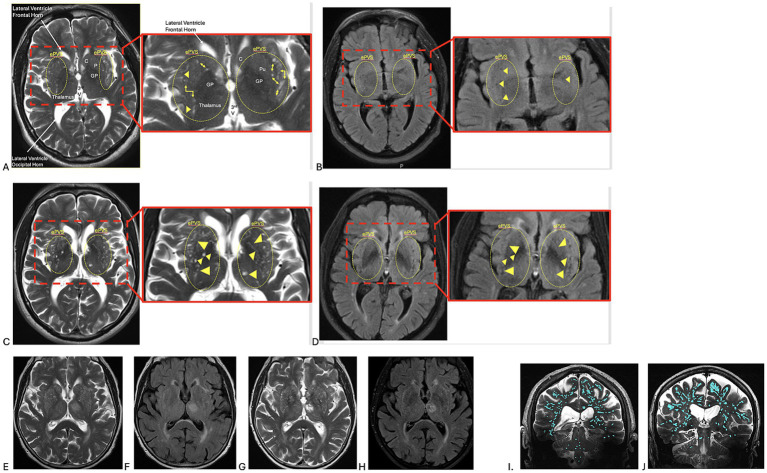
(A,B) Longitudinal MRI of a 65-year-old male individual with Alzheimer’s dementia on 1.5 T MRI. (A) Axial T2 and (B) axial FLAIR images at baseline. A total of 10 years later, the same patient is shown on (C) axial T2 and (D) axial FLAIR images, now demonstrating enlarged perivascular spaces (ePVSs) (highlighted within yellow dotted lines and indicated by yellow arrows and arrowheads) in the basal ganglia and periventricular white matter. The ePVSs are markedly enlarged on scans obtained 10 years later (C,D). They appear hyperintense on T2-weighted sequences with fluid content and hypointense on FLAIR sequences. In contrast, white matter microvascular disease, unlike PVSs, is hyperintense on both T2- and FLAIR-weighted sequences. AC, anterior commissure; ACA, anterior cerebral artery; C, caudate; ePVS, Enlarged perivascular space; F, fornix; GP, globus pallidus; MCA, middle cerebral artery; MTT, mammillothalamic tract; PC, posterior commissure; PCFF, posterior commissural fibers of the fornix; PVS, perivascular space. (E–H) An 85-year-old female individual with vascular dementia on 3 T brain MRI. (E,F) Axial T2-weighted and FLAIR images demonstrate multiple foci of white matter hyperintensity, likely sequelae of microvascular disease, along with ePVSs in the lentiform nuclei, thalami, and periventricular white matter, exhibiting an “etat crible” appearance. A total of 2 months later, after contracting COVID-19, the same patient is shown in (G,H), demonstrating persistent white matter microvascular disease along with increased size of multiple ePVSs in the lentiform nuclei, thalami, and periventricular white matter. (I,J) Ultra-high field 7 T MRI coronal T2-weighted images, with enlarged perivascular spaces (ePVSs) highlighted in light blue: (I) a healthy control and (J) a similarly aged patient with mild cognitive impairment demonstrating prominent ePVSs.
